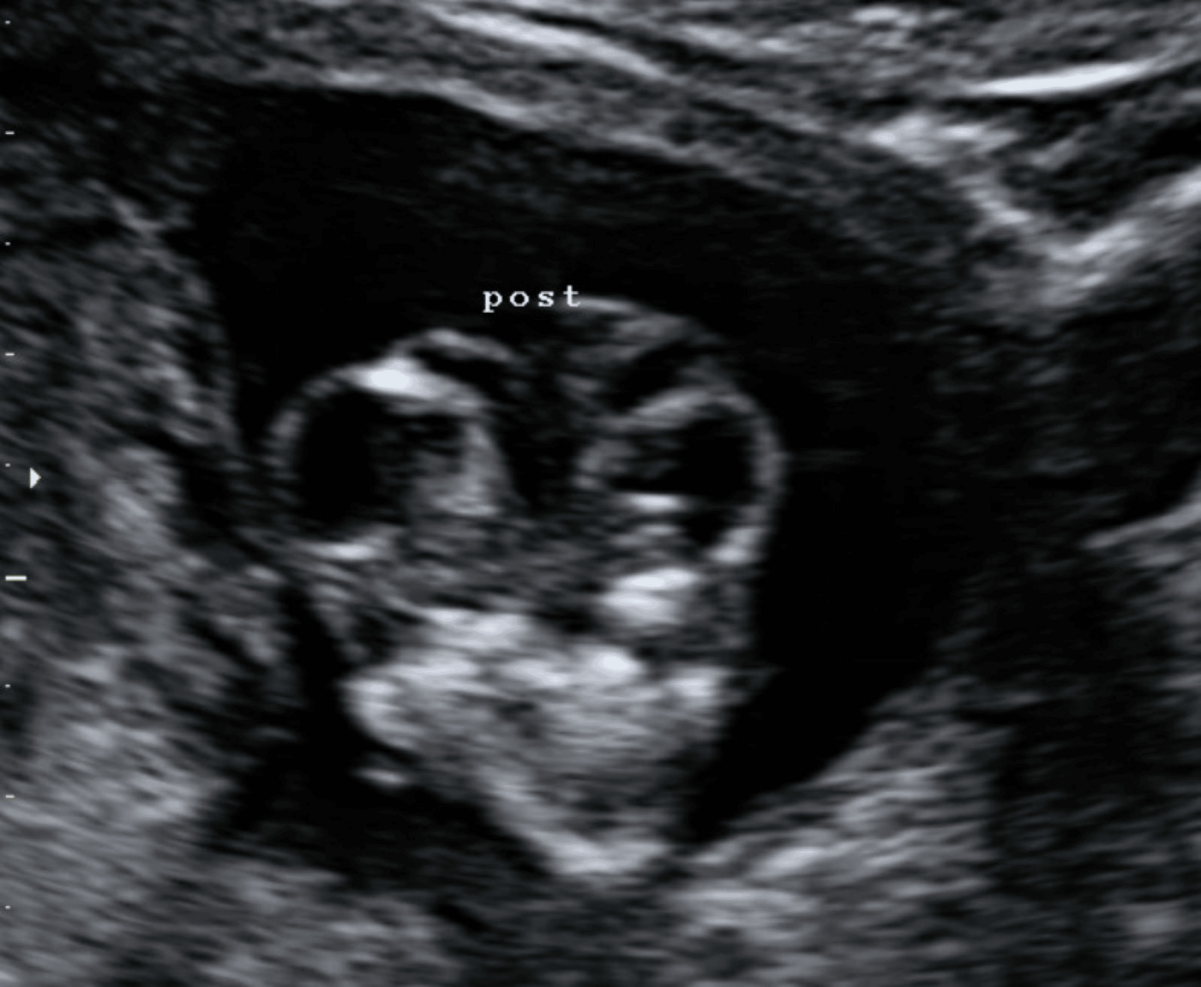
Transverse cervical section of the fetus in the ultrasound at 12 weeks and six days of gestation representing an open neural tube defect
